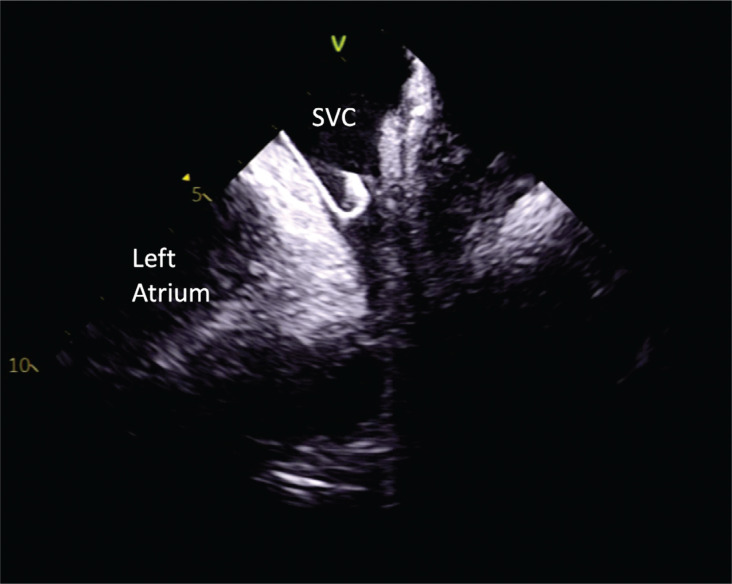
Intracardiac echocardiography image showing the J-tip wire in the superior vena cava. *Abbreviation:* SVC, superior vena cava.
